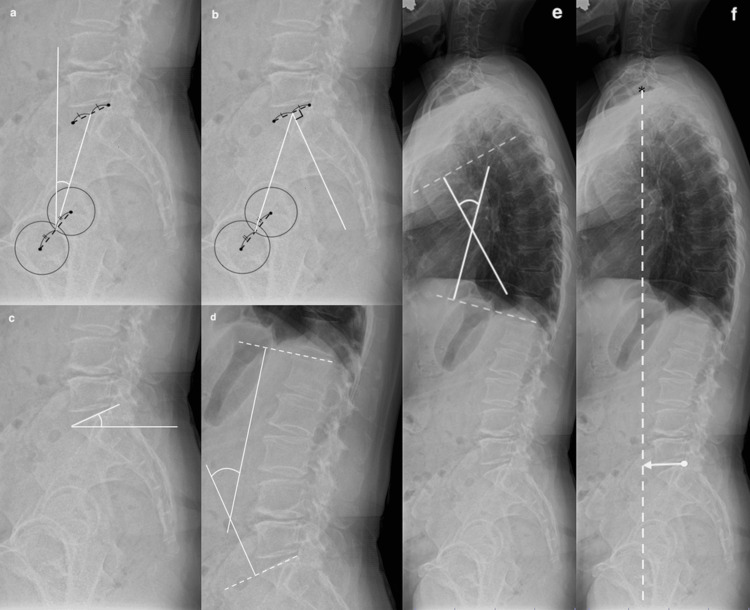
Radiographic parameters on whole-spine standing lateral radiographs (a) PT: The angle between the line from the center of the femoral heads to the midpoint of the S1 endplate and the vertical line. (b) PI: The angle between the line connecting the midpoint of the S1 endplate to the center of the femoral heads and the lines perpendicular to the S1 endplate. (c) SS: The angle between the superior endplate of S1 and the horizontal line. (d) LL: The angle between the lines perpendicular to the superior endplates of L1 and S1. (e) TK: The angle between the lines perpendicular to the superior endplate of T4 and the inferior endplate of T12. (f) SVA: The horizontal distance between the vertical line from the center of C7 and the posterosuperior corner of the S1 endplate (the asterisk indicates the center of the seventh cervical vertebra). PT: Pelvic tilt, PI: Pelvic incidence, SS: Sacral slope, LL: Lumbar lordosis, TK: Thoracic kyphosis, SVA: Sagittal vertical axis Image credit: Author Tetsuro Shimada
